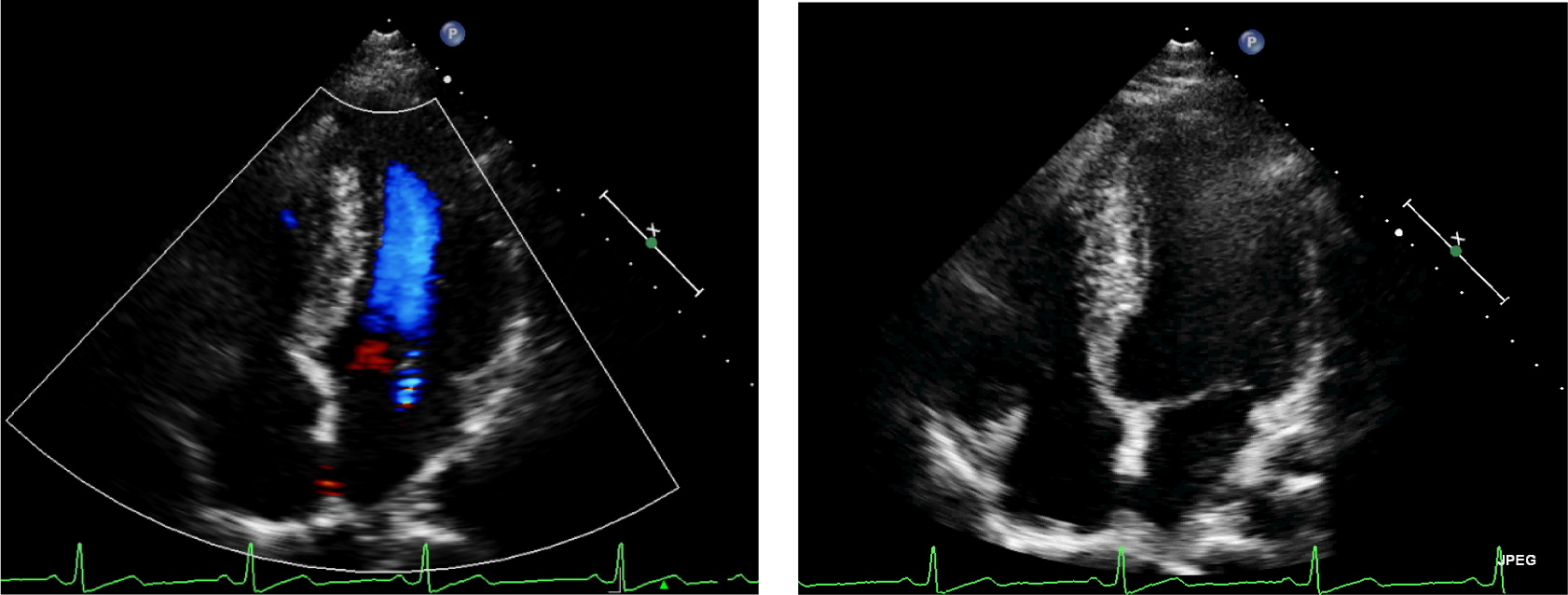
Cardiac Echocardiography showed asymmetric left ventricular hypertrophy with diastolic dysfunction
